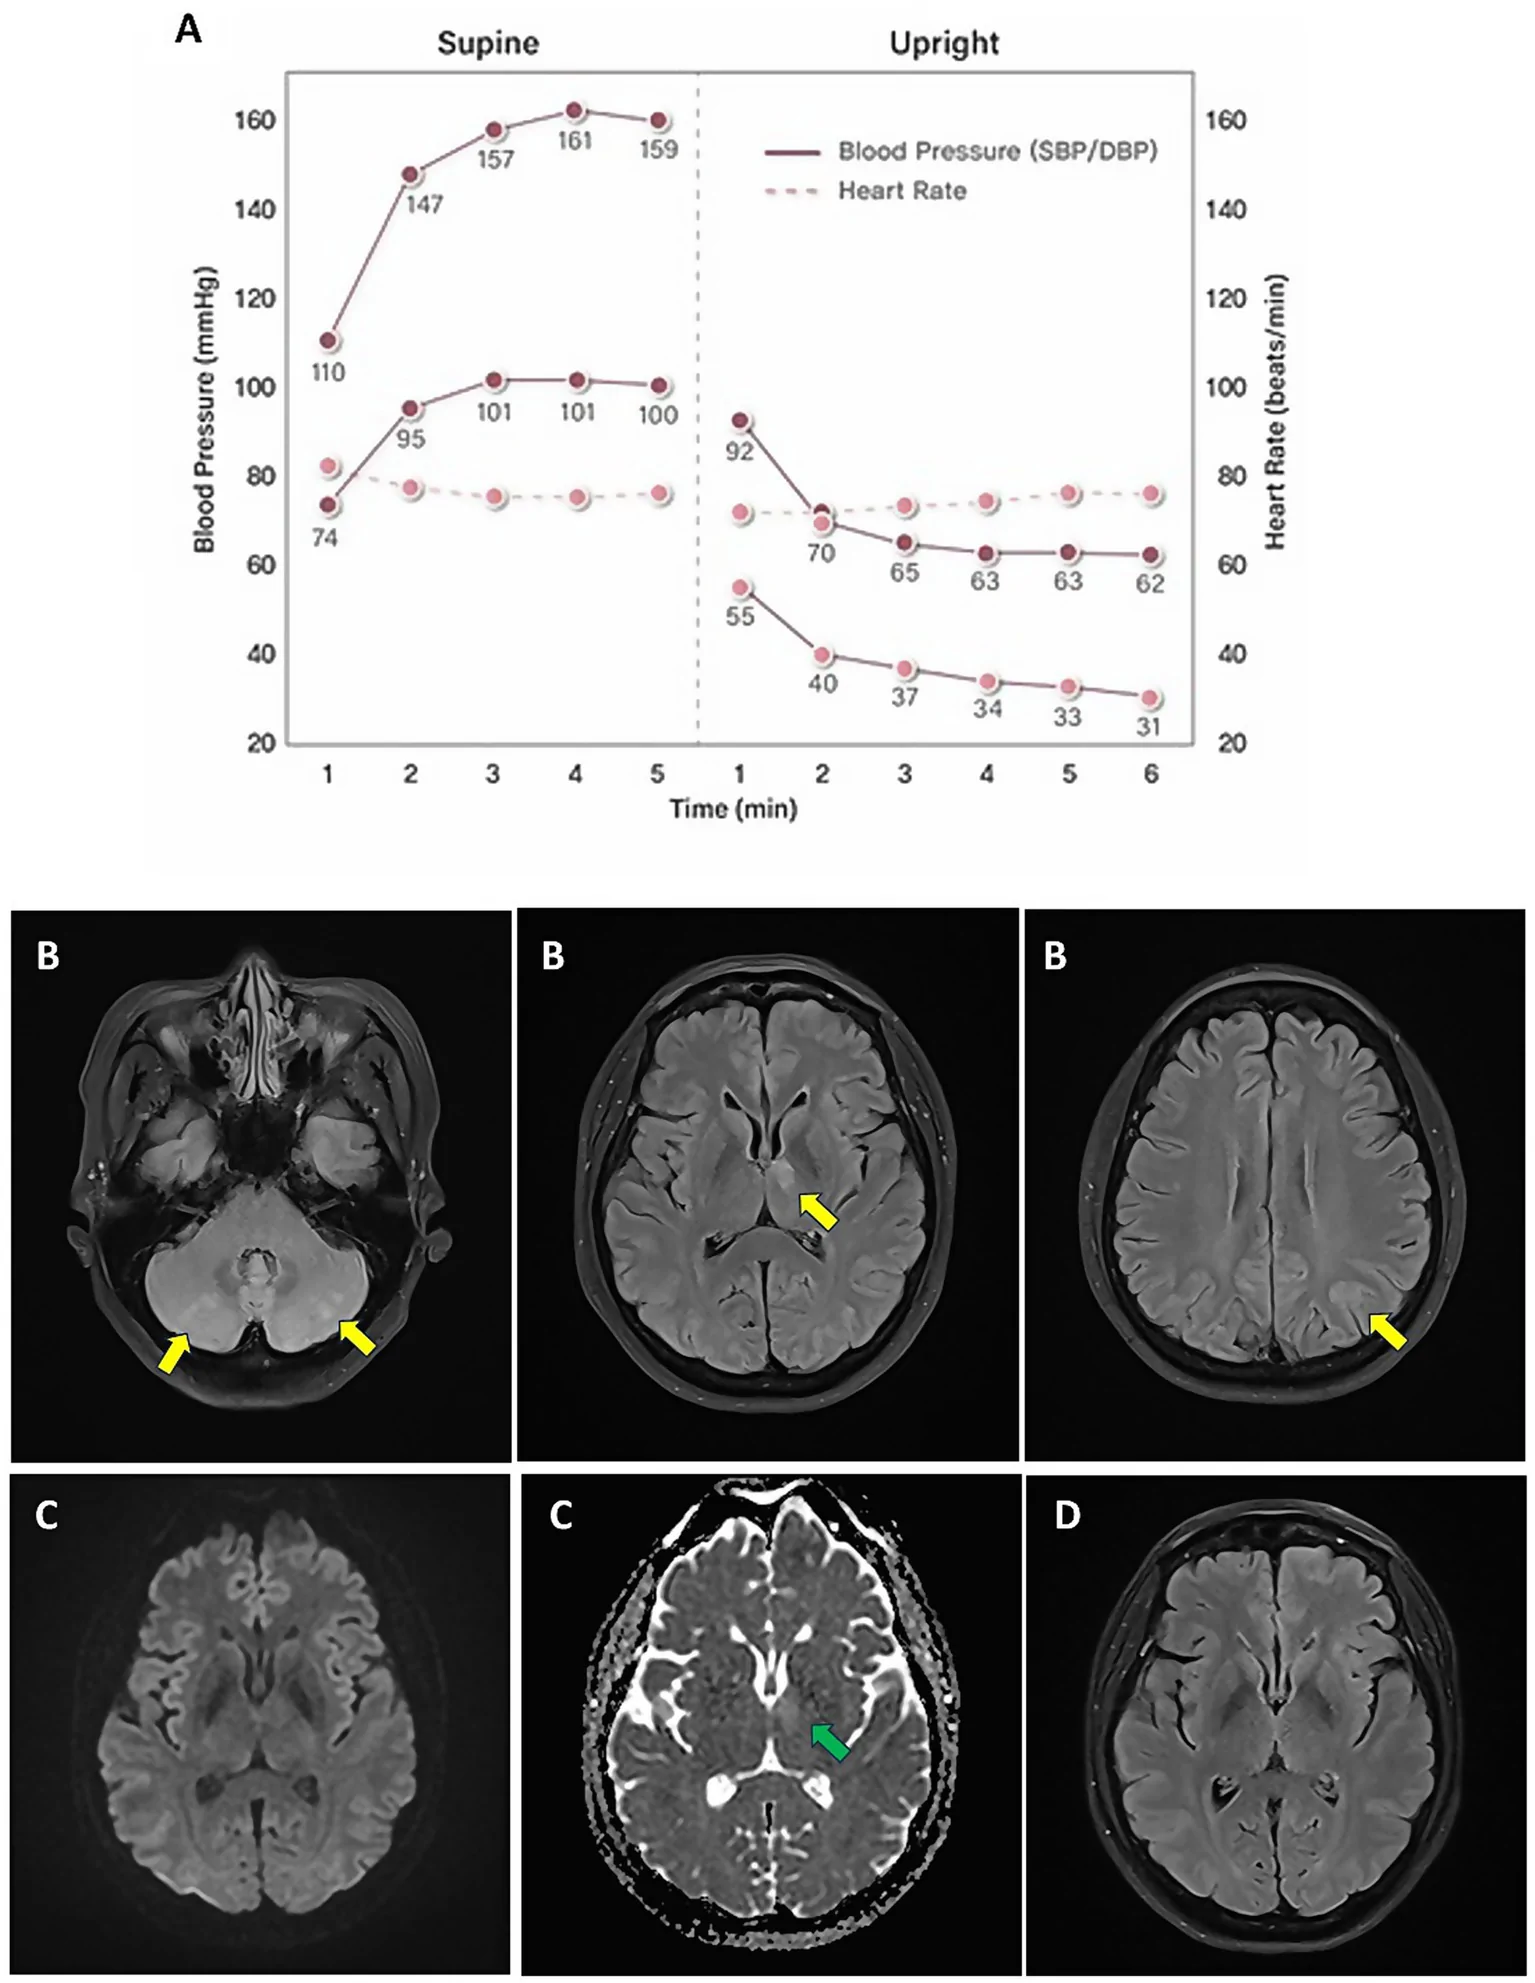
Tilt table test and brain MRI findings. (A) During the supine phase, blood pressure was initially within the normal range but gradually increased to the hypertensive range. Upon tilting upright, the patient exhibited a marked drop in systolic and diastolic blood pressure without a compensatory increase in heart rate. The test was terminated at 6 min because of severe dizziness. (B) Axial fluid-attenuated inversion recovery (FLAIR) MR image demonstrates multifocal hyperintense lesions involving both cerebellar hemispheres, the left medial thalamus, and the parietal region (yellow arrows). (C) Diffusion-weighted imaging (DWI) showed no diffusion restriction, and apparent diffusion coefficient (ADC) maps demonstrated mildly increased signal intensity (green arrow). (D) Follow-up FLAIR MR image obtained one week later demonstrated complete resolution of the previously noted lesions.
